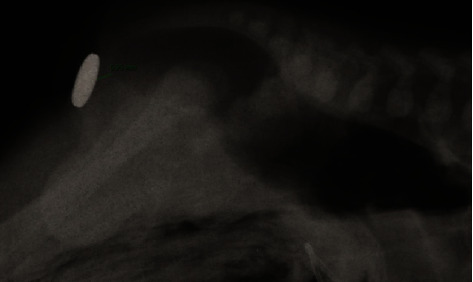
Cross-table lateral radiograph taken at 24 hours of life. A radiopaque marker was placed at the level of the anal dimple.
